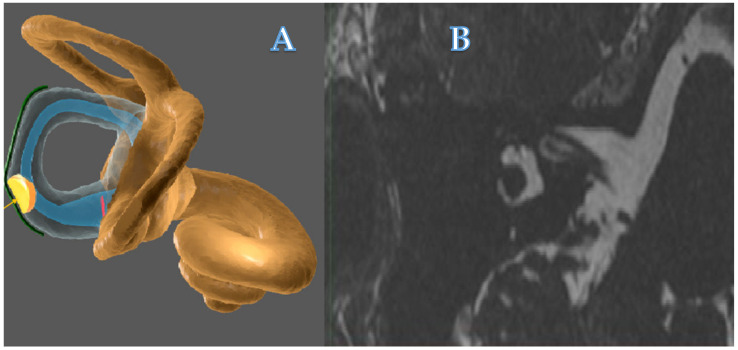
(A) Conceptual imaging of HSCC bone plugging. (B) MRI T2 picture of postsurgical state with interrupted HSCC. Right labyrinth.
